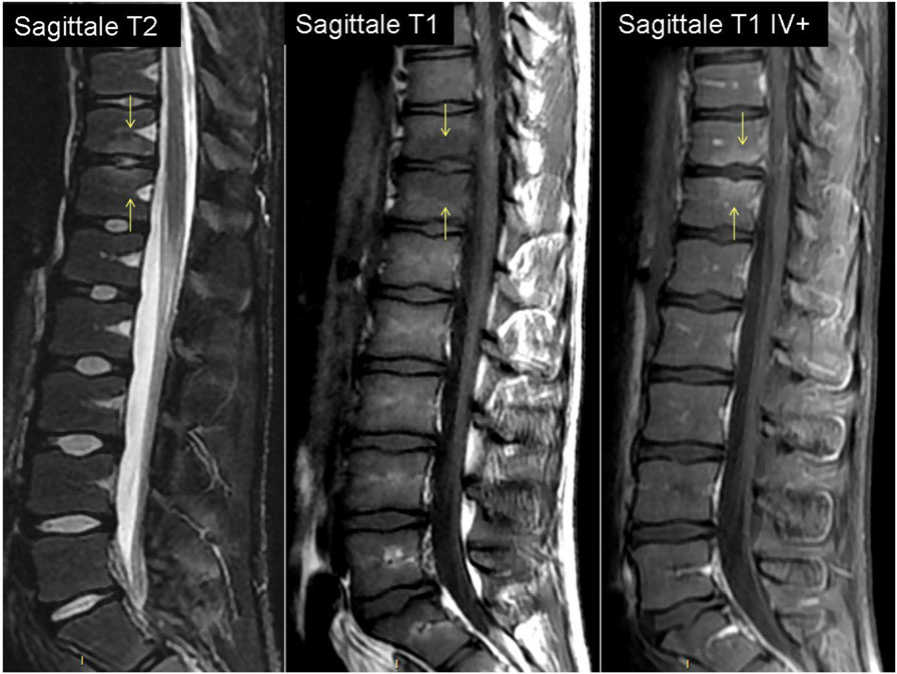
Magnetic resonance imaging of the spine showing a T11-T12 spondylodiscitis. Sagittal T2-weighted, T1-weighted and contrast-enhanced T1-weighted sequences demonstrate mirror oedema (hypersignal T2, hyposignal T1 enhancing after contrast injection) of vertebral adjacent endplates (inferior endplate of T11 and superior endplate of T12) surrounding the intervertebral disk T11-T12, which has decreased T2 signal. There is no soft tissue or epidural extension of the inflammation. With courtesy of Dr. S. Lamer (CH Courbevoie-Neuilly)
